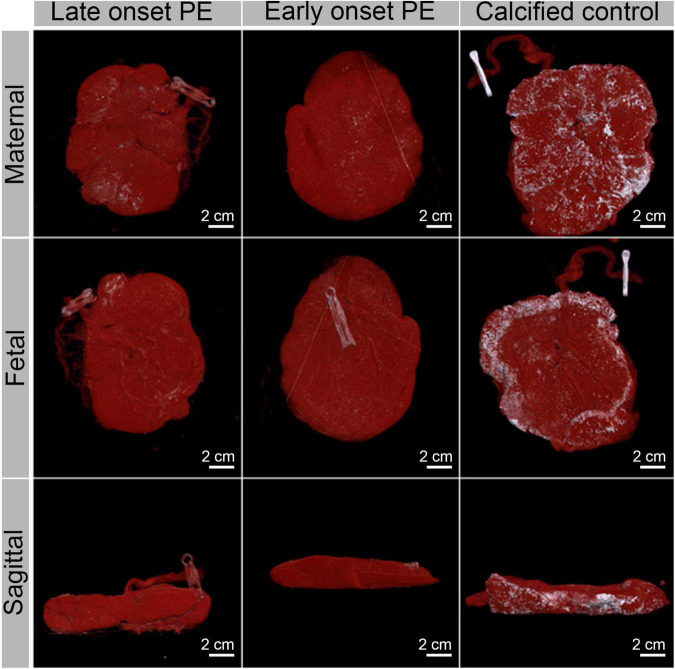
Computed tomography images of three representative placentae (a late onset PE placenta, an early onset PE placenta and a calcified control placenta, see Table 2 for their characteristics) in maternal, fetal and sagittal view. Soft tissue is shown in red while calcifications appear in white.
